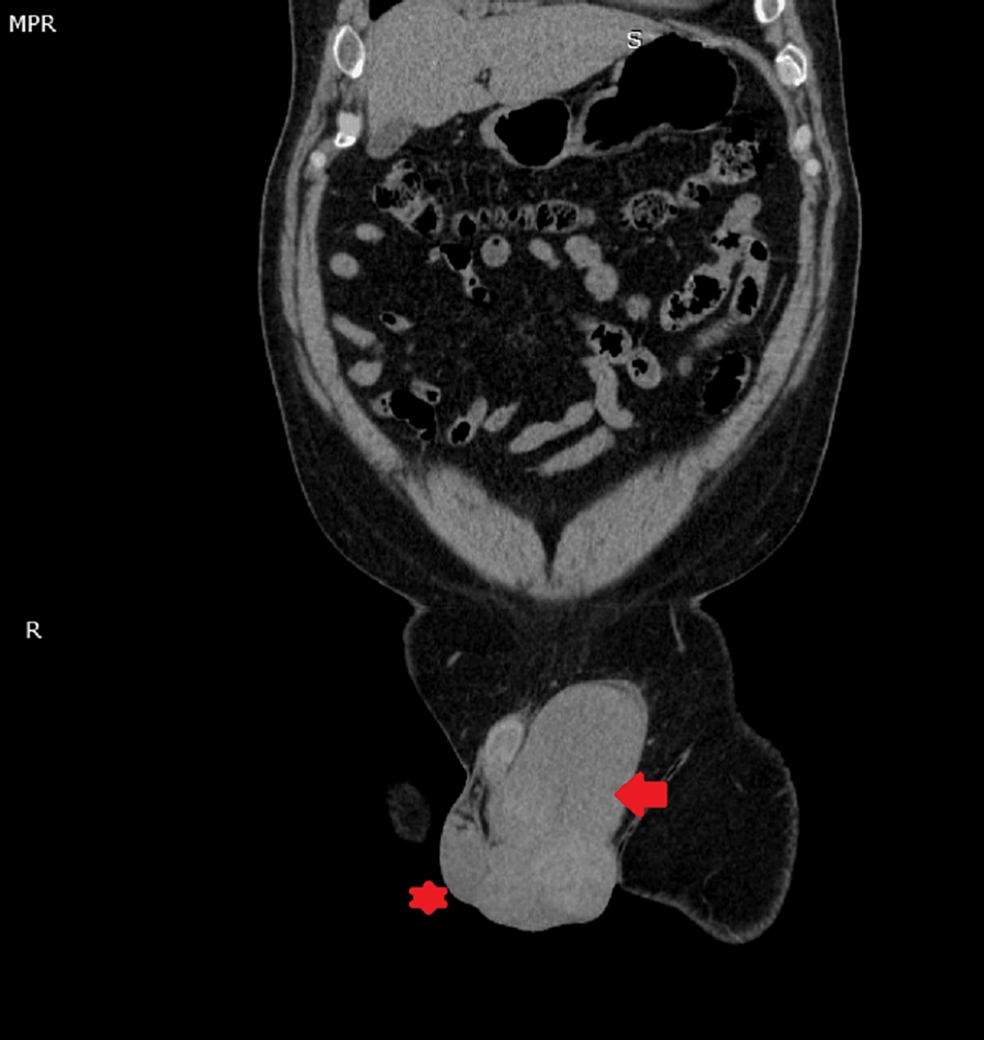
(Asterix symbol) marks the location of the normal testis on the right of the patient, and the → (arrow head) symbol marks the location of the affected testis on the left of the patient. Scan showed is a coronal section of a non-contrast CT of the patient.
